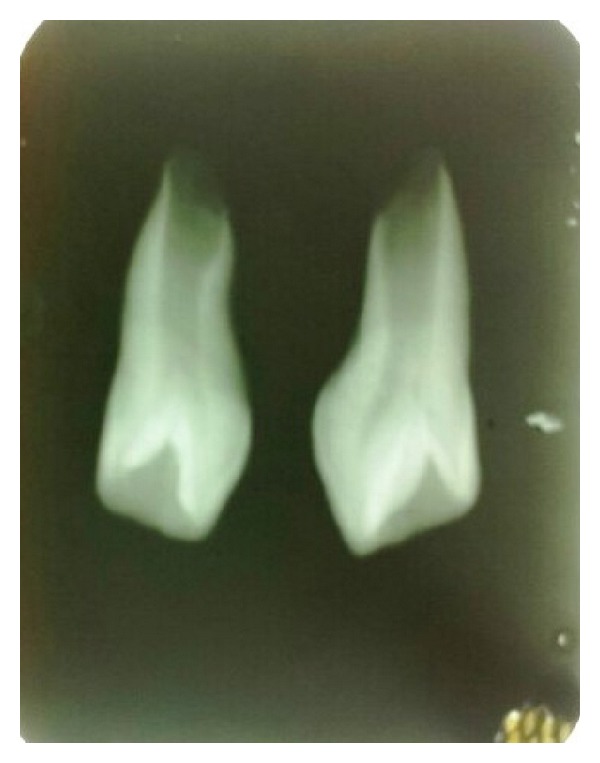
Postoperative X-ray showing incomplete root formation of mesiodens.
